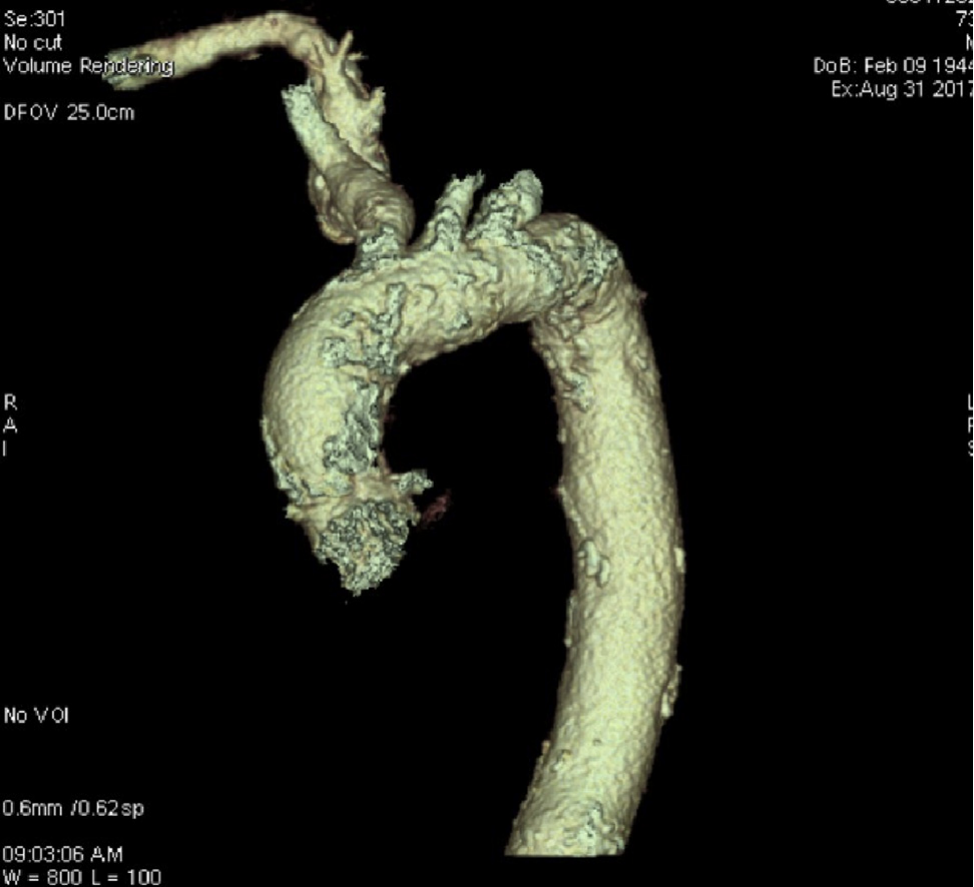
Computed tomography scan reconstruction showing the severe calcification of the ascending aorta, the arch, and head vessels.
